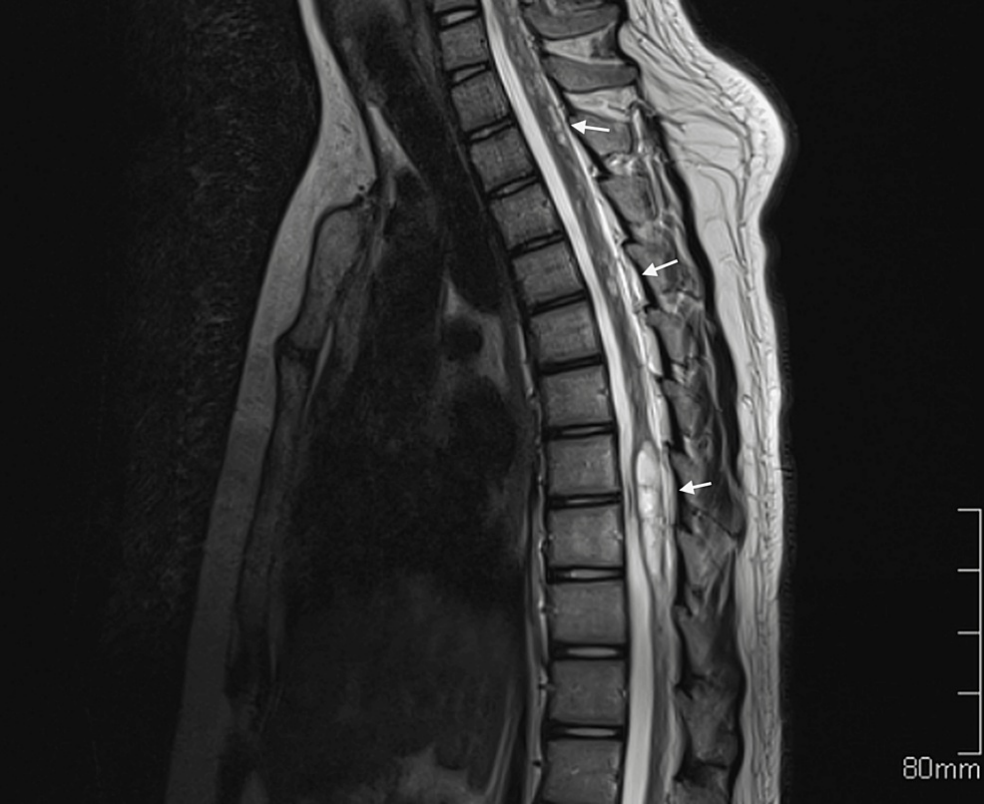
Sagittal T2-weighted spinal imaging showing multiple cystic lesions of varying sizes (arrows)
